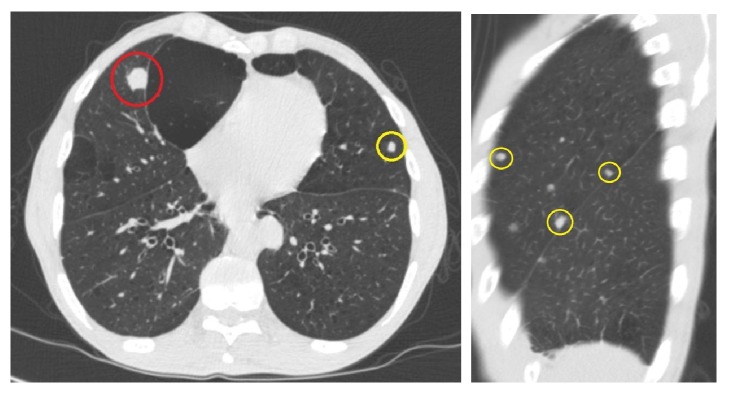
Noncontrast axial and sagittal CT images of the chest show interval enlargement of prior right middle lobe nodule (red circle) and multiple new nodules throughout the lungs (yellow circles).
